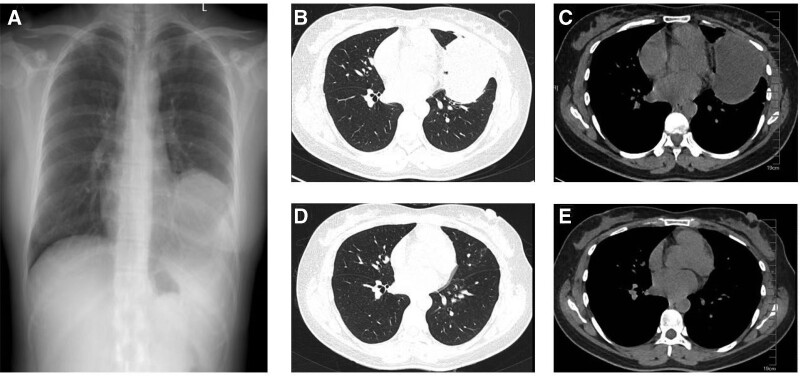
(A–C) CT scans of the chest showed multiple encapsulated effusions adjacent to the left ventricle in the left costophrenic angle. (D and E) Follow-up chest CT scans. CT = computed tomography.
